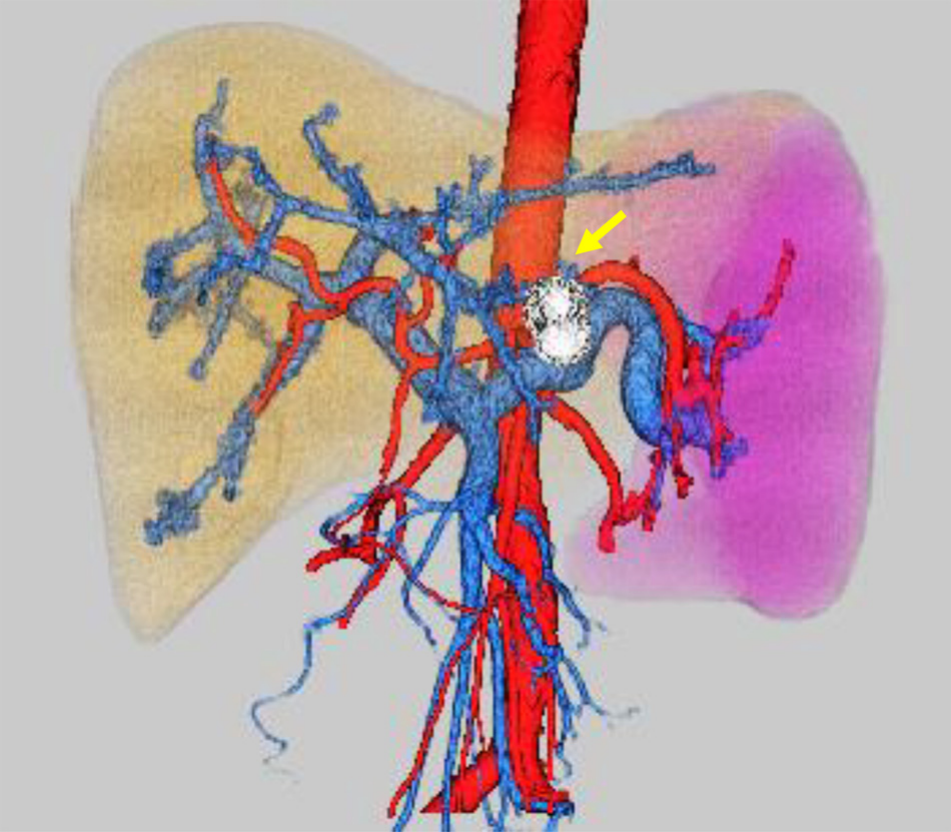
3D-CT reconstruction image. 3D-CT reconstruction image shows hepatosplenomegaly and increased splenic vein diameter. The spleen volume is 1011 mL and the liver volume is 2461 mL, respectively. Spleen/liver volume ratio is 0.41. No development of portal collaterals is observed. The tumor (arrow) is located in segment 3. CT, computed tomography.
